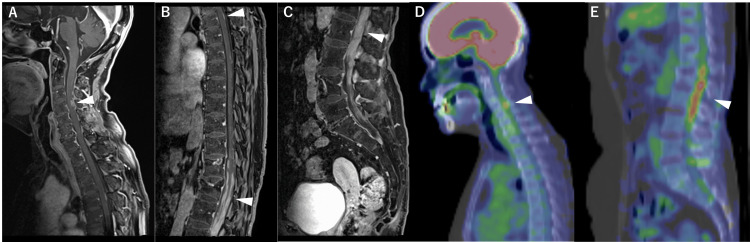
Images of spinal dissemination Whole spine Gd T1-weighted MRI shows enhanced mass lesions (arrowheads) at C3-4 (A), T4 (B), and cauda equina (C). FDG-PET shows accumulations in the same lesions (D, E).
